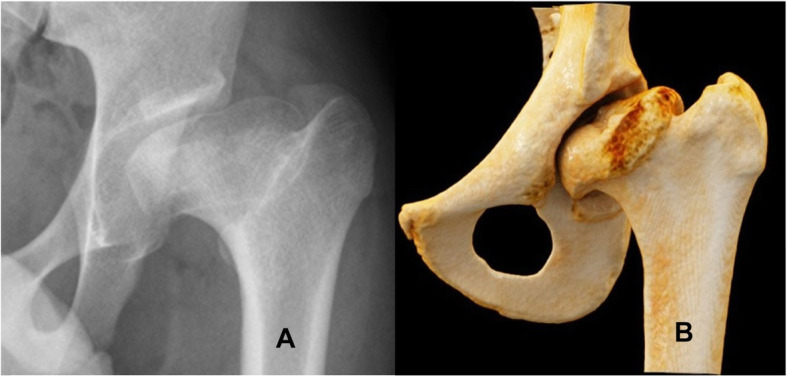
Radiographs (a) and volumetric rendering (b) of a hip affected by LCP disease. The new contour of the head resembles a mushroom with a short neck and has a high-riding trochanter
